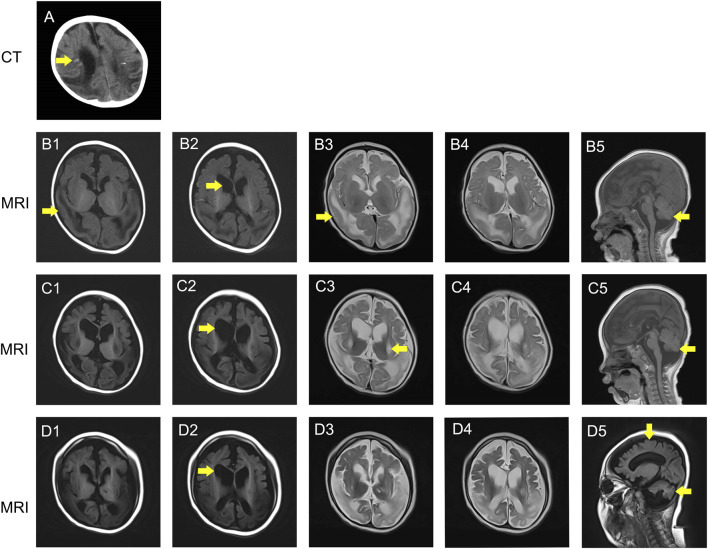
Neuroimaging results of the patient. (A) CT result of the brain (7 months of age): Small irregular calcifications next to the lateral ventricle. (B1-D5) MRI result of the brain. ((B1-B5) 3 months old, (C1-C5) 1.25 years old, (D1-D5) 4 years old): The MRI showed pachygyria in the temporal lobes (B1, B3). Brain MRI performed at 3 months, 1 year, and 3 months, and 4 years of age revealed progressive whole brain atrophy (B5, C5, and D5) respectively).
